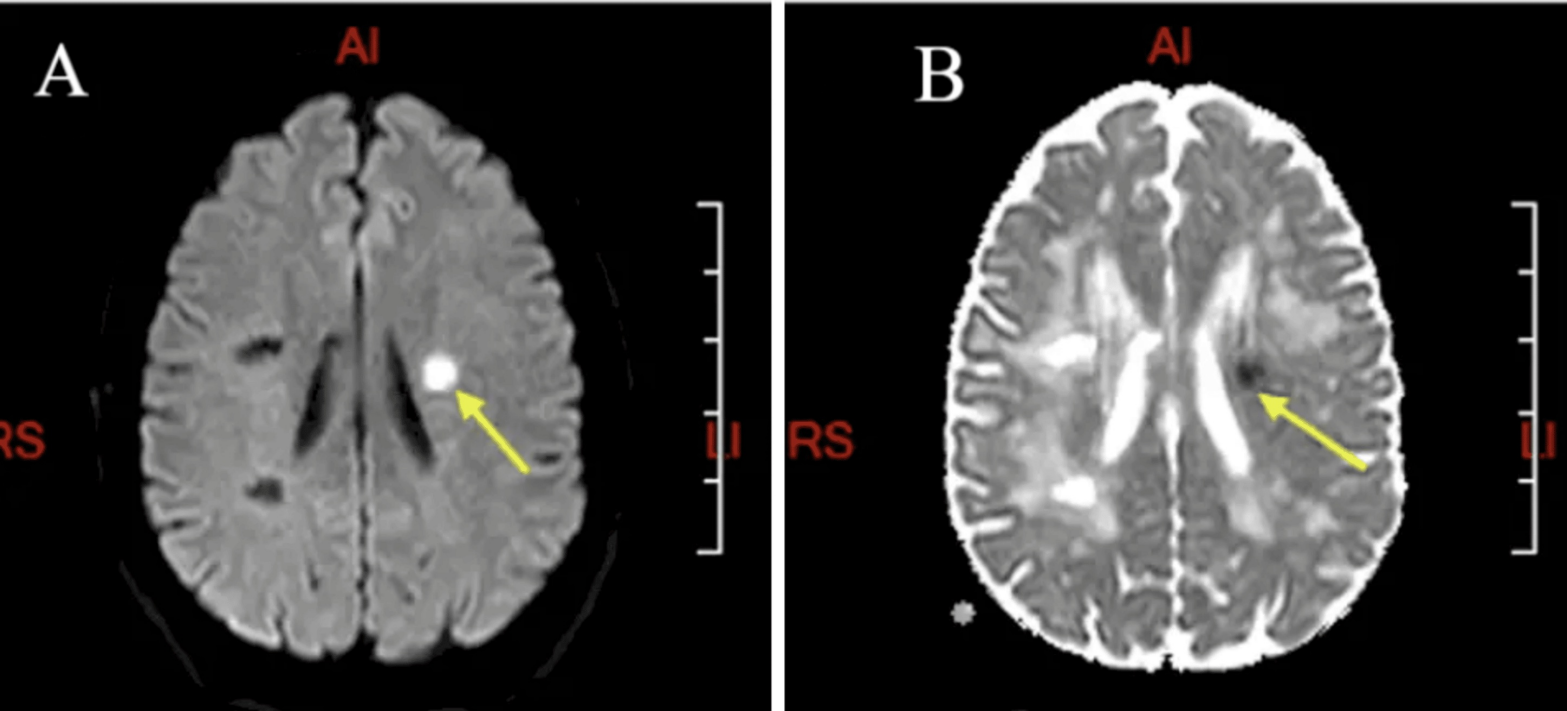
Brain MRI of the index patient: DWI and ADC sequences DWI hyperintense lesion (yellow arrow) (A), with corresponding ADC lesion (yellow arrow) (B) suggestive of acute ischaemic stroke DWI: diffusion-weighted imaging; ADC: apparent diffusion coefficient
